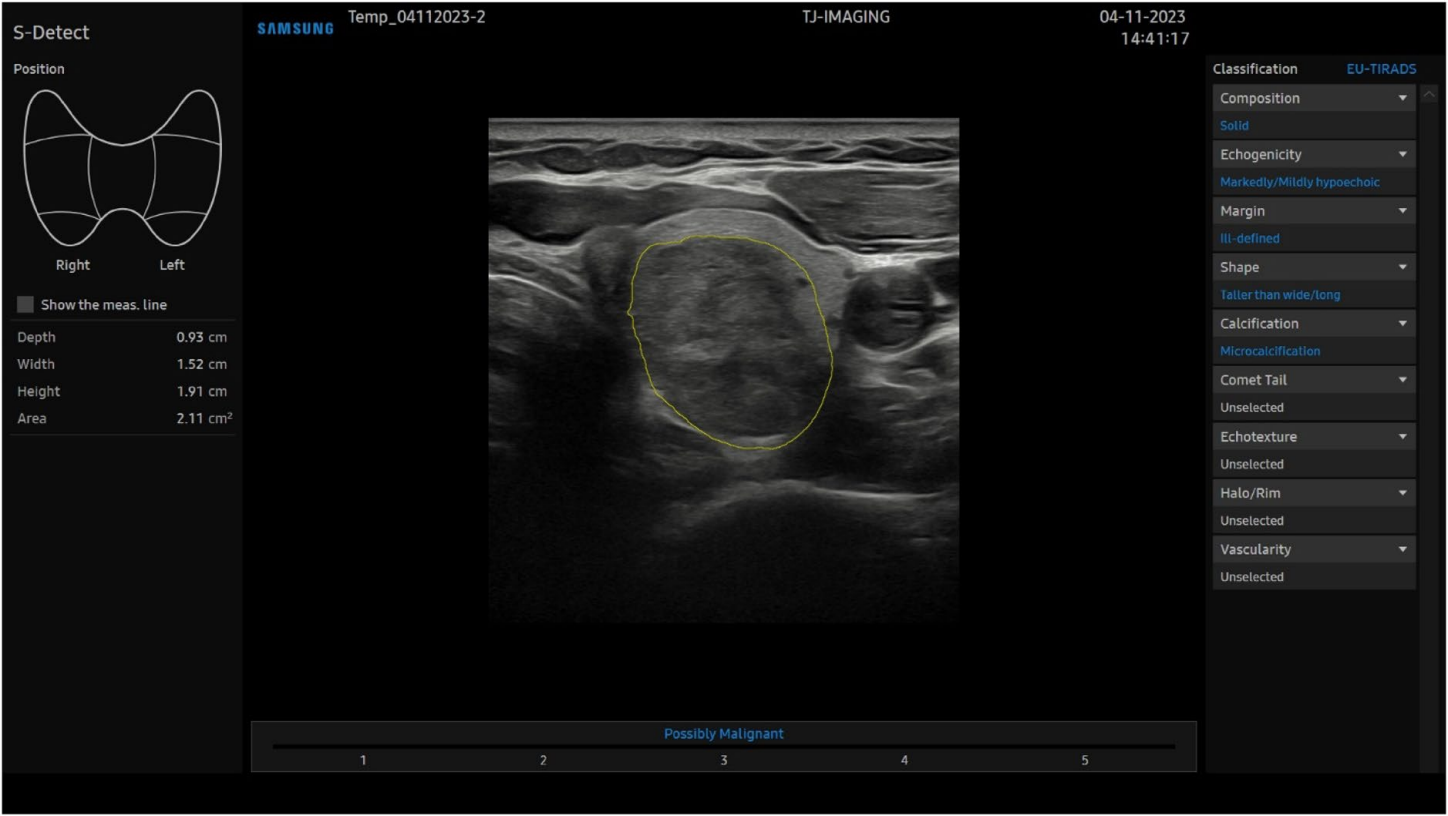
Greyscale US transverse image of a left thyroid lobe with S-Detect’s analysis of a thyroid nodule. Nodule measurements are on the left, nodule classification in EU-TIRADS on the right, and the diagnosis as “Possibly Malignant” on the bottom. US = Ultrasound. S-Detect = Artificial intelligence-based program for diagnosing thyroid nodules. EU-TIRADS = European Thyroid Imaging Reporting And Data System
